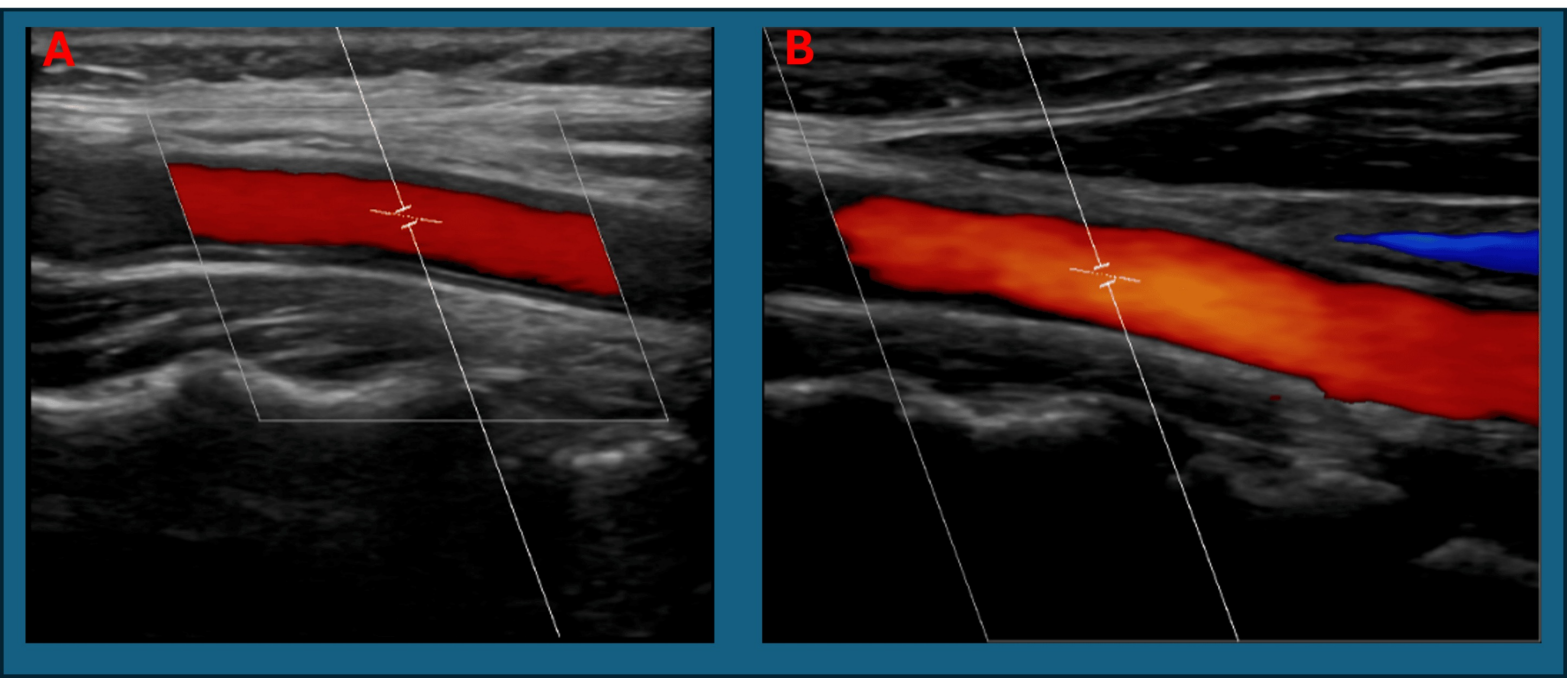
A: Normal ultrasound carotid Doppler of right common carotid artery (peak systolic velocity: 112.2 cm/s, end-diastolic velocity: 22.4 cm/s). B: Normal ultrasound carotid Doppler of left common carotid artery (peak systolic velocity: 129.0 cm/s, end-diastolic velocity: 19.5 cm/s)
